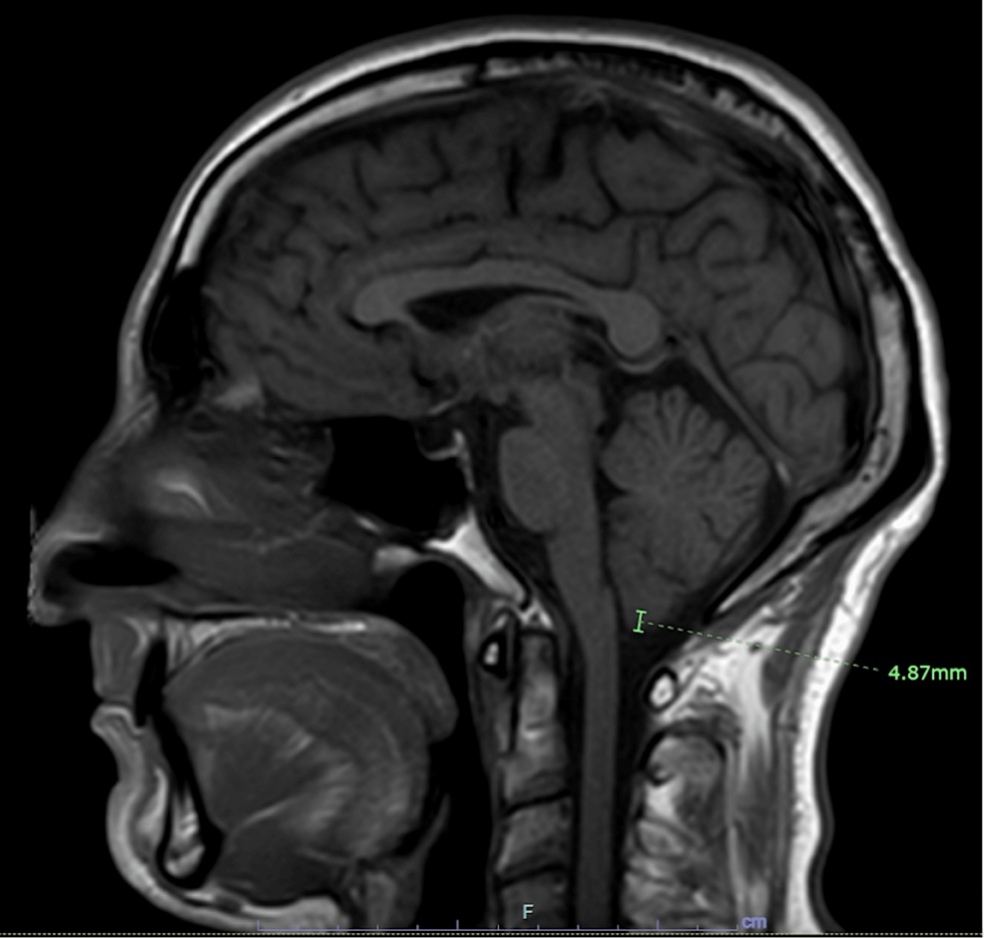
Non-Contrast Enhanced Sagittal T1-weighted Magnetic Resonance Image (MRI) of the Brain Demonstrates 4.87 mm Downward Herniation of the Cerebellar Tonsils into the Foramen Magnum
